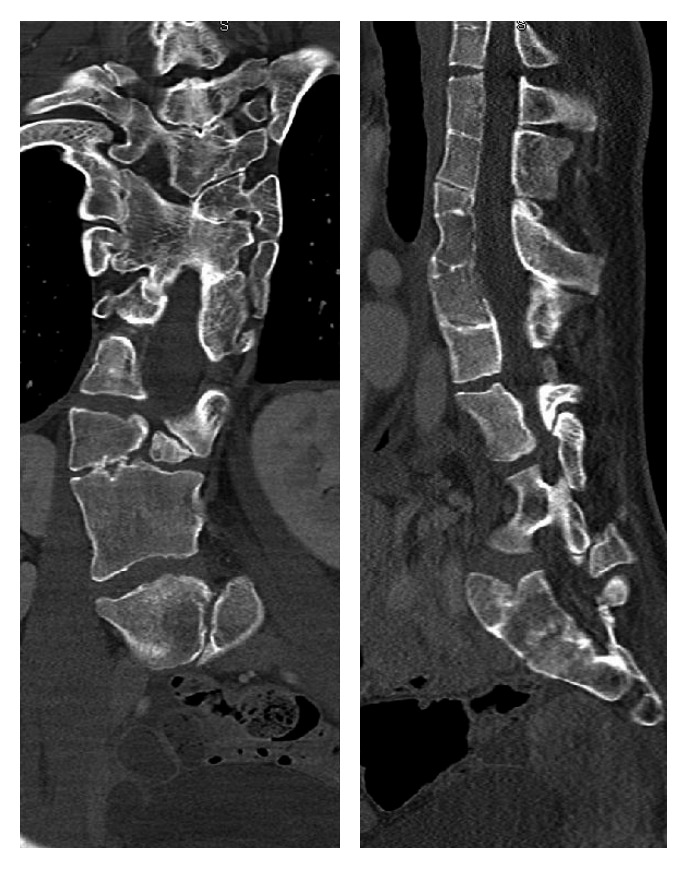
Coronal and sagittal CT scan of the thoracolumbar spine and sacrum demonstrating a variety of vertebral segmentation defects including hemi-, wedge-, butterfly-, and block vertebra.
